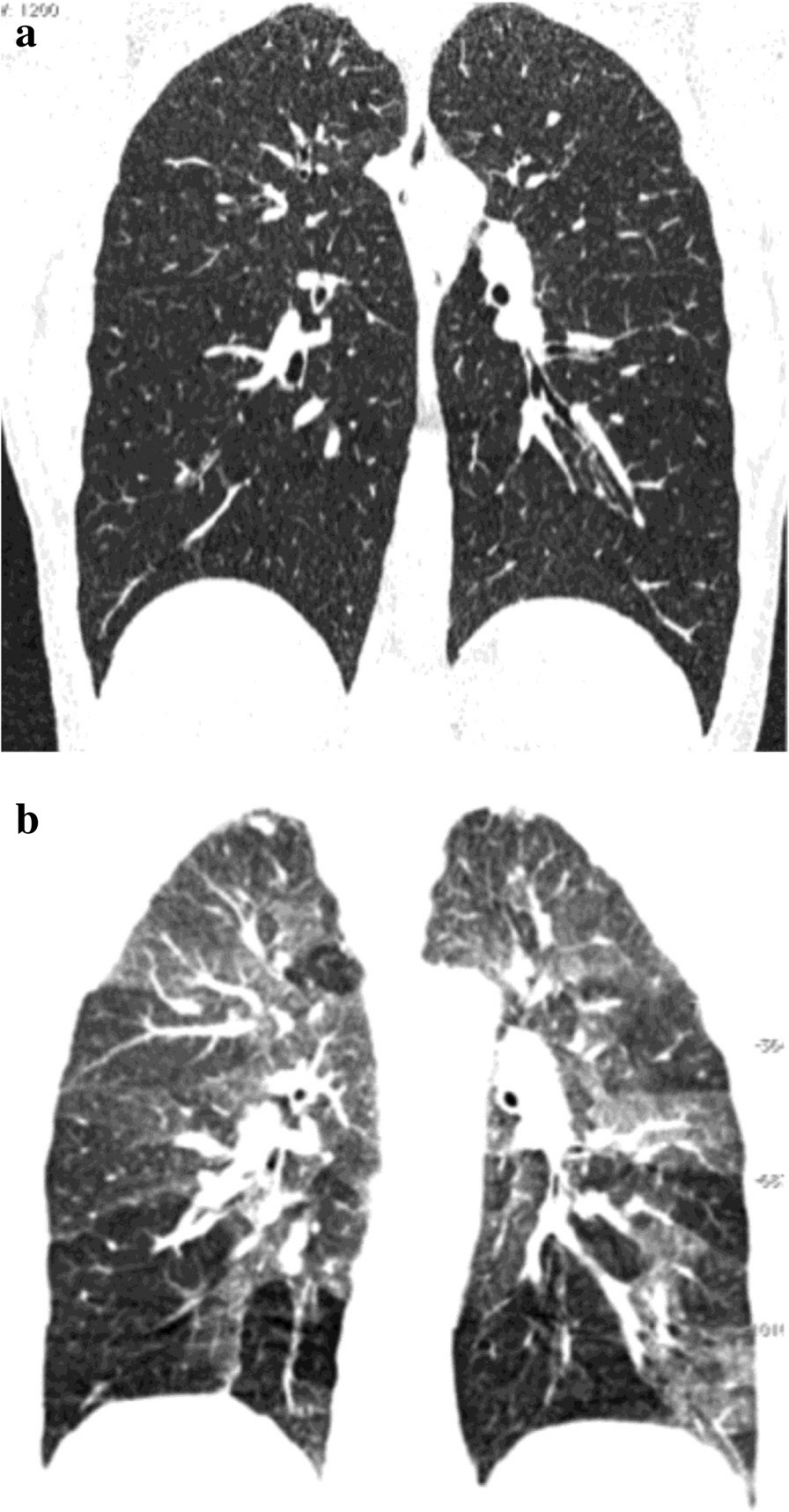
23-year-old male with mushroom worker’s lung disease referred for slight exertional dyspnea and dry cough after two acute respiratory attacks. On coronally-reformatted inspiratory CT image no lung abnormalities can be seen (a). Coronally-reformatted expiratory CT image shows extensive air-trapping (b). Diagnosis was confirmed by bronchoalveolar lavage.
